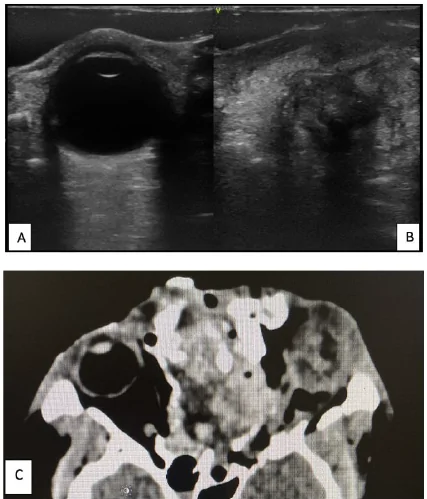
Case 1. Modified water bath technique used in a patient globe rupture: A) normal right eye; B) globe rupture of left eye; and C) computed tomography suggestive of left globe rupture.
